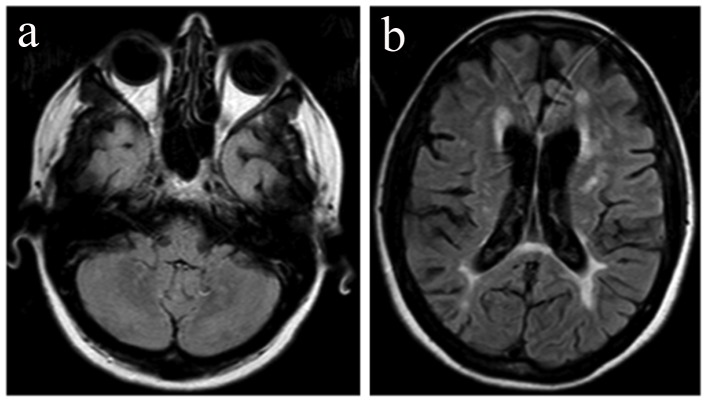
On a follow-up MRI scan 12 months after the initial relapse, FLAIR images reveal complete resolution of the signal abnormalities in the left cerebellar hemisphere (a) and in the right parietal lobe (b).
